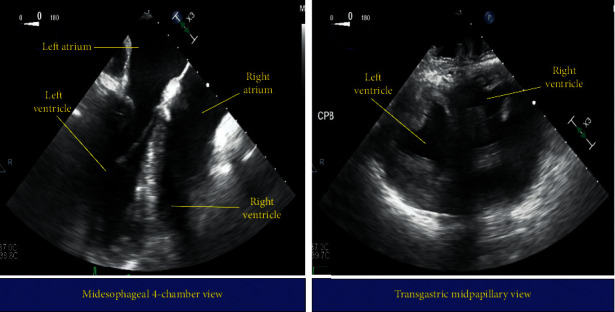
Midesophageal 4-chamber (left) and transgastric midpapillary transesophageal (right) echocardiography images.
